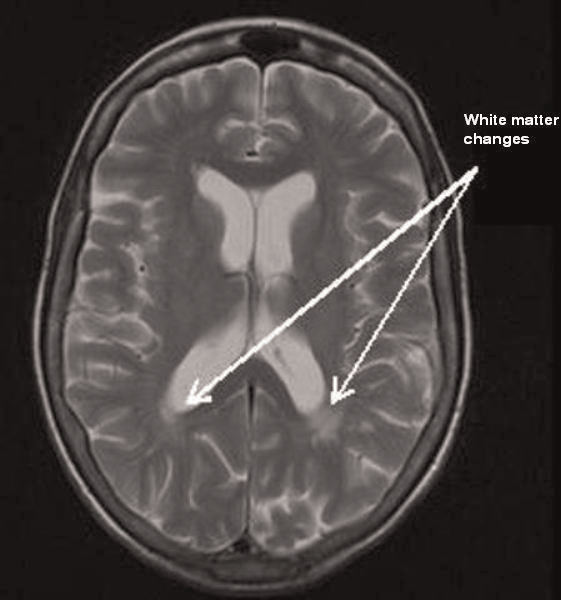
Magnetic Resonance Imaging showing white matter changes in the parietal area post gadolinium enhancement.
